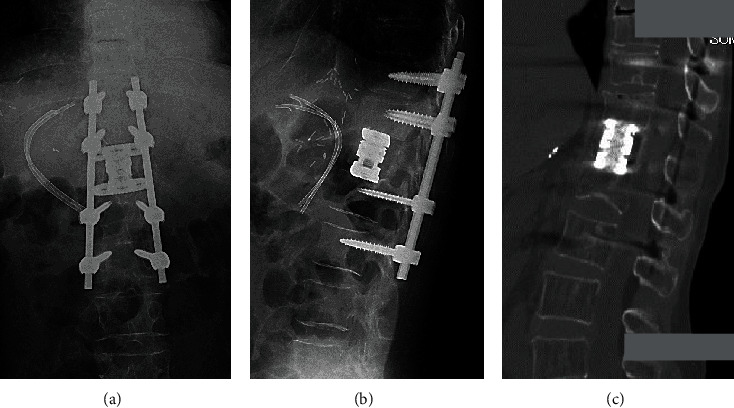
Postoperative radiographs and computed tomography (CT) images of the thoracolumbar spine of a 66-year-old woman with collapse of the 12^th^ thoracic (T12) vertebra. (a) anteroposterior view X-ray; (b) lateral view X-ray; (c) sagittal CT image two years following surgery.
